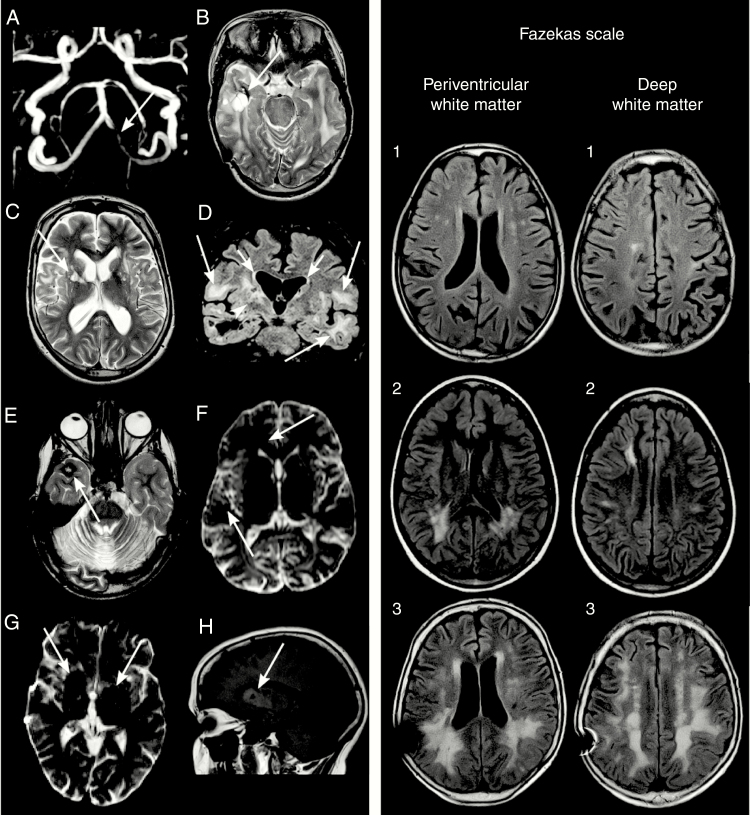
Panel 1, A to D, A, arrow, A 27-year-old male patient had 50% stenosis of the intracranial left vertebral artery on MRA after a follow-up of 21 years. T2-weighted axial images show a B, arrow, 3-cm cavity of hyperintense fluid with a surrounding hypointense hemosiderin rim and septations located in the right temporal lobe indicative of radiation necrosis and a C, arrow, hyperintense lacunar infarct on the right basal ganglia. D, A T2 fluid-attenuated inversion recovery coronal image demonstrated a large confluent of deep white matter (WM) (Fazekas grade 3, long arrows) and moderate periventricular (Fazekas grade 2, short arrows) hyperintensities, suggesting severe vasculopathy. D, arrowhead, The right basal ganglia were surrounded by a T2-hyperintense area, indicating vasculopathy. Panel 1, E to F, E, arrow, A 24-year-old male patient had a cavernoma in the right temporal lobe defined by the presence of a typical hyperintense nidus and a dark rim of hemosiderin on a T2-weighted axial image with no surrounding cerebral edema. F, arrows, On diffusion-weighted imaging, several small, rounded hypointense microbleeds were detected in the right cerebral hemisphere. MRI was performed 17 years after radiotherapy. Panel 1, G to H, G, arrows, A 31-year-old female patient had bilateral hypointense lesions on the basal ganglia on diffusion-weighted imaging that showed a heterogeneous signal on H, arrow, T1-weighted sagittal images typical of calcifications. The follow-up time of the female patient was 23 years. Panel 2 demonstrates representative T2 fluid-attenuated inversion recovery images of mild (grade 1), moderate (grade 2), and severe (grade 3) cerebral WM hyperintensities classified using the Fazekas scale (Table 1).^26^ A 41-year-old male patient showed multiple, small, punctate deep WM Fazekas grade 1 hyperintensities after a 23-year follow-up. A 19-year-old female patient demonstrated after a follow-up time of 11 years several periventricular and deep WM Fazekas grade 2 hyperintensities. A 43-year-old male patient showed large confluent periventricular and deep WM Fazekas grade 3 hyperintensities after a follow-time of 26 years.
